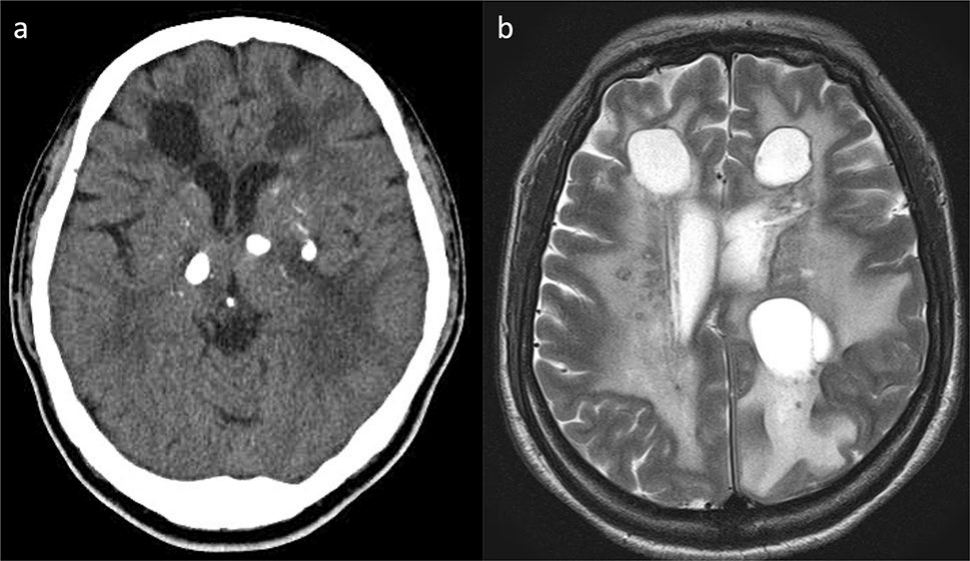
Leukoencephalopathy with calcifications and cysts (LCC) in a 45-year-old, female patient with headache, cognitive decline, spasticity, and speech disturbance. a Axial non-contrast computed tomography revealed multiple, asymmetrical calcifications in the bilateral basal ganglia, thalami, and subcortical white matter and cyst formation in the bilateral, frontal white matter. b T2-weighted imaging demonstrated multiple, giant cysts and leukoencephalopathy, along with multiple, punctate hypointensities reflecting the calcifications in the bilateral white matter (this case was provided by professor Junichi Takanashi of Tokyo Women’s Medical University, Yachiyo Medical Center)
